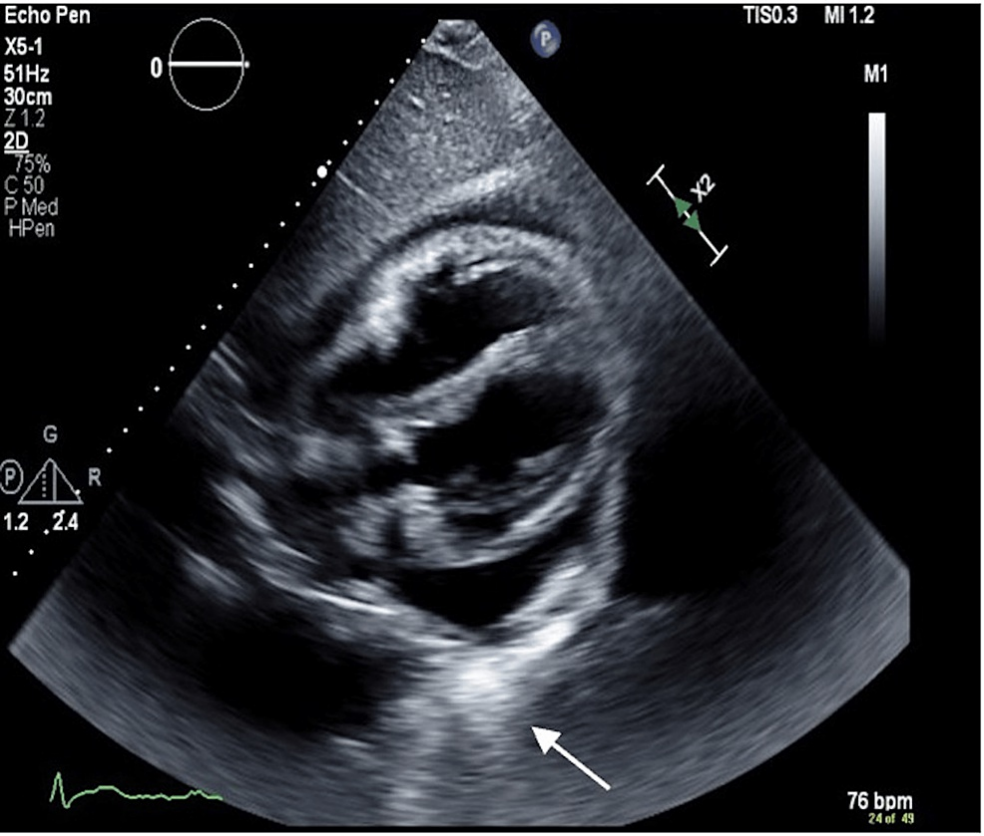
Echocardiogram subcostal four-chamber view (white arrow is pointing to pericardial effusion)
